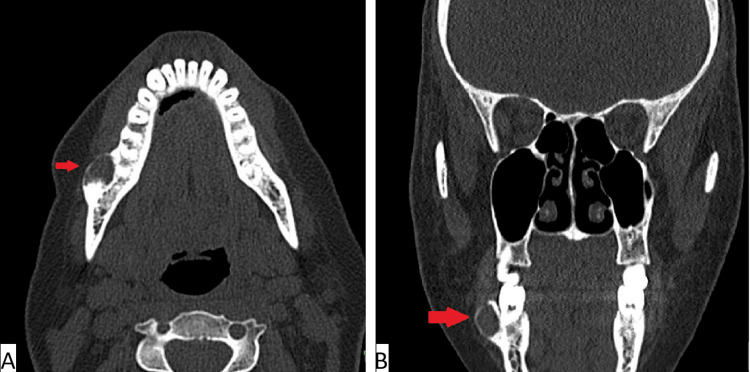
Noncontrast computed tomography photograph of maxillofacial bones demonstrating an oval, intra-osseous, unilocular, lytic lesion with sclerotic margins (red arrows), located posterolateral to the last right molar tooth. A: axial view;  B: coronal view.
